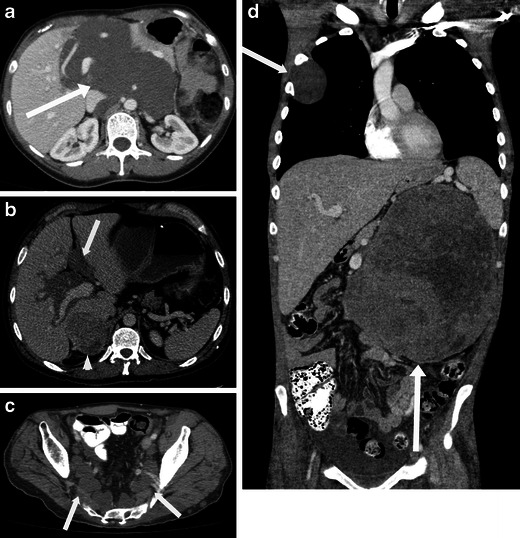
Imaging in neurofibromatosis (NF-1). a Plexiform neurofibromatosis in a 36-year-old woman with NF-1. Axial contrast enhanced image from upper abdomen shows a large lobulated hypodense mass (*arrow*) encasing the vessels without causing any narrowing. The adjacent organs are displaced by the central mass without invasion. b Plexiform neurofibromatosis and pheochromocytoma in a 45-year-old man with NF-1. Contrast enhanced CT scan shows an ill-defined hypodense plexiform neurofibromatosis mass (*arrow*) extending along the portal triad in the liver. The heterogeneously enhancing right adrenal mass (*arrowhead*) was a pheochromocytoma on resection. c, d A 29-year-old man with NF-1. Multiple low density well-defined hypodense neurofibromas along the sacral plexus were seen in the pelvis on both sides (*arrows*) on CT scan (c). On a follow up CT scan (d), a large new heterogeneous solid mass (*arrow*) was seen in left retroperitnoeum, proven to be a malignant peripheral nerve sheath tumour on resection. A neurofibroma is also seen in the right chest (*arrow*)
